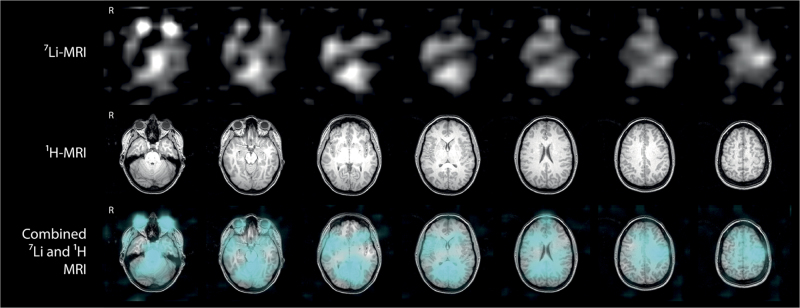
^7^Li b-SSFP MRI in a single subject. The ^7^Li-MRI dataset (upper row) was acquired in 8 min and is presented with corresponding ^1^H-MRI axial images (middle row). The combined images (lower row) show ^7^Li b-SSFP MRI (in cyan) overlaid on ^1^H-MRI images. The distribution of lithium in the brain is heterogeneous and the greatest signal intensity arises from the orbits
